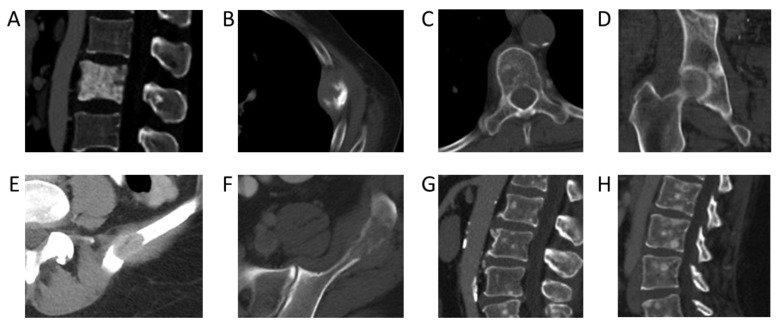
Bone metastases from primary cancers with the highest incidence rates. (A) Sclerotic pelvic metastases in a 76-year-old male patient with prostate cancer. (B) Left 5th rib lytic metastasis in an 84-year-old male with colorectal cancer. (C) Lytic metastasis T5 vertebra in a 64-year-old female patient with ovarian cancer. (D) Sclerotic metastasis right acetabulum in a 62-year-old male patient with urothelial cancer. (E) Lytic metastasis left ilium in a 54-year-old female patient with renal cell cancer. (F) Lytic metastasis left ilium in a 44-year-old male patient with renal cell carcinoma. (G) Multiple sclerotic lumbar spine metastases in a 54-year-old male patient with urothelial carcinoma of the renal pelvis. (H) Sagittal CT lumbar spine of multifocal sclerotic osseous metastases in a 35-year-old female patient with HER2-negative gastric cancer.
